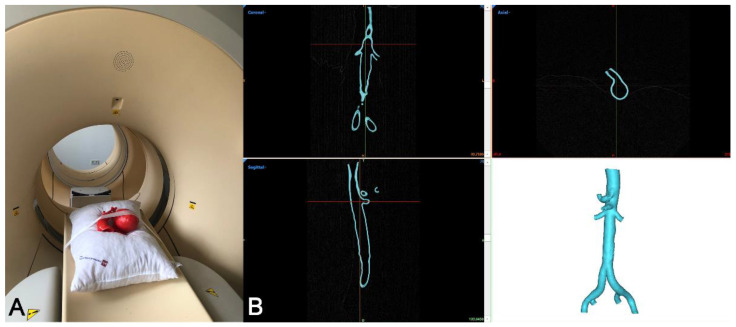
CT scanning of the 3D-printed model. (A): A high-resolution CT scan (slice thickness 0.4 mm) was obtained from all 35 3D-printed models. (B): The 3D model was segmented using MIS, and an STL file (3D model STL) was generated.
